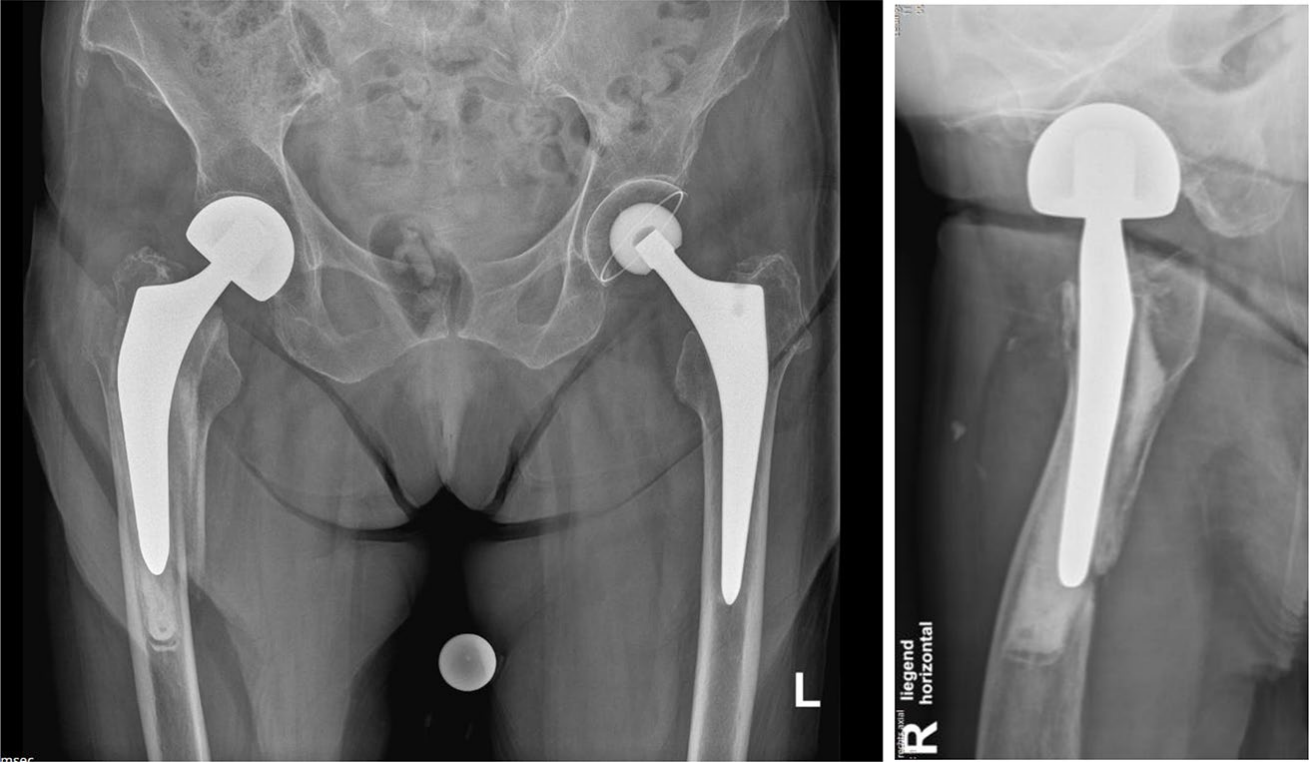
Radiograph of a right-sided Vancouver B2 fracture around a cemented polished stem. Anteroposterior view and lateral view
